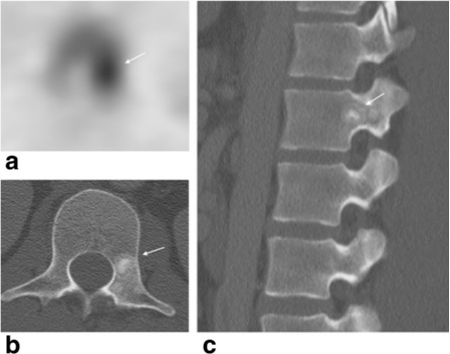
Osteoid osteoma lesion at L1 pedicle with “double intensity sign” on bone scan. a Axial SPECT image show focal increased uptake in the left pedicle with a surrounding area of less intense uptake (“double intensity sign“). b Axial CT image of L1 vertebra and c sagittal image of the spine (between T12 and L3 levels) show a sclerotic lesion at L1 left pedicle with a central lucency which is almost completely calcified
